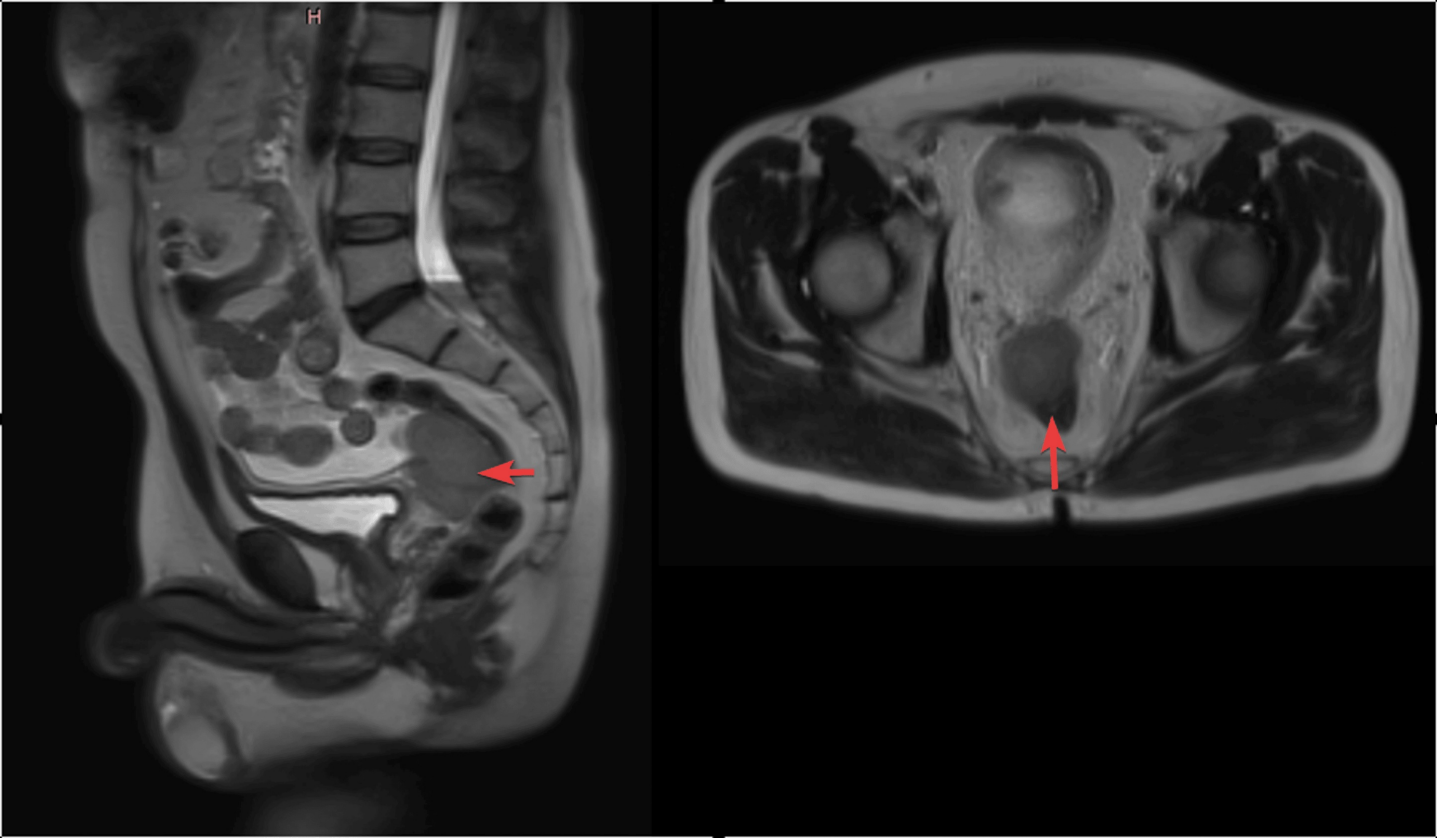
The MRI of the pelvis with contrast (August 5, 2024) revealed a soft tissue lesion in the rectovesical space, potentially indicative of a peritoneal or serosal deposit. The arrows indicate a soft tissue lesion in the rectovesical space; the lesion was external to the rectum and rectosigmoid junction.
